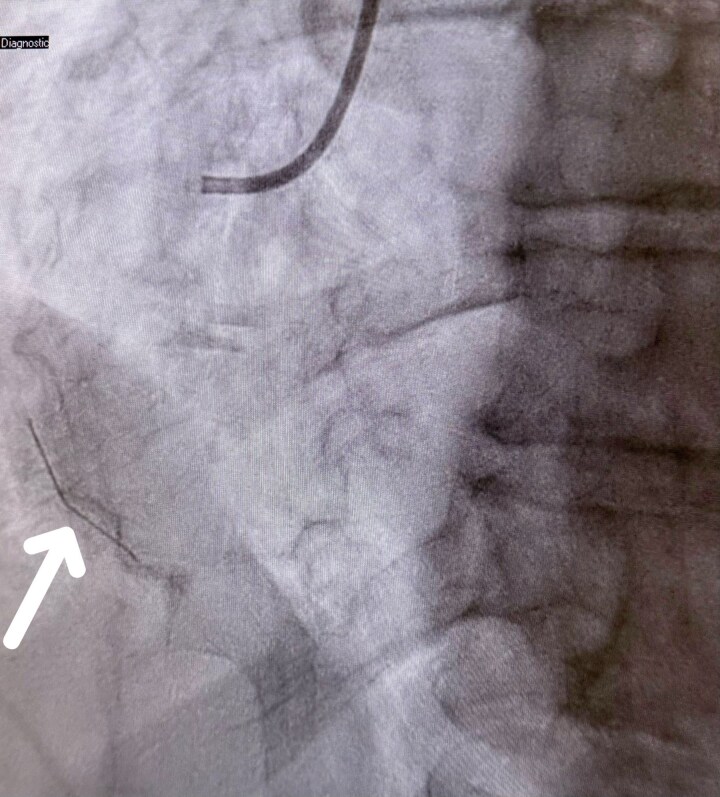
Abdominal radiograph (kidney–ureters–bladder view). Plain abdominal radiograph showing fractured and displaced components of the inferior vena cava filter (arrow), with absence of one limb. Radiographic configuration is most consistent with a retrievable BARD G2 filter design.
